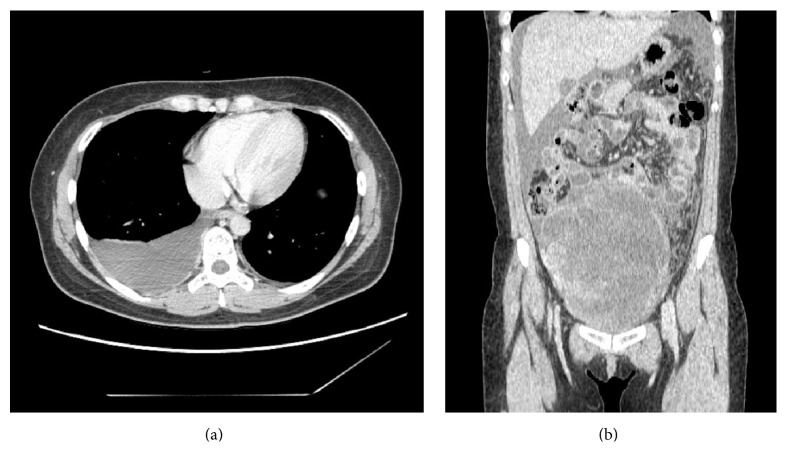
Contrast-enhanced CT scan in case 3 shows pleural effusion in the right thorax ((a) axial plane) and ascites along with a well-circumscribed and heterogeneously enhanced tumor of the rt. ovary ((b) coronal plane).
